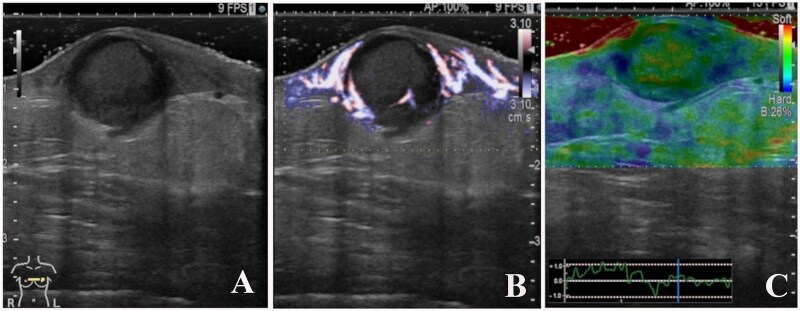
B-mode ultrasound imaging revealed that the nodule had an ill-defined, oval-shaped, and demonstrated dorsal acoustic amplification and lateral shadowing and heterogeneous pattern with a hypoechoic rim (A). Color doppler sonography showed blood flow signals mainly around the nodule (B), and on ultrasound elastography, the nodule appeared green-to-orange (C).
